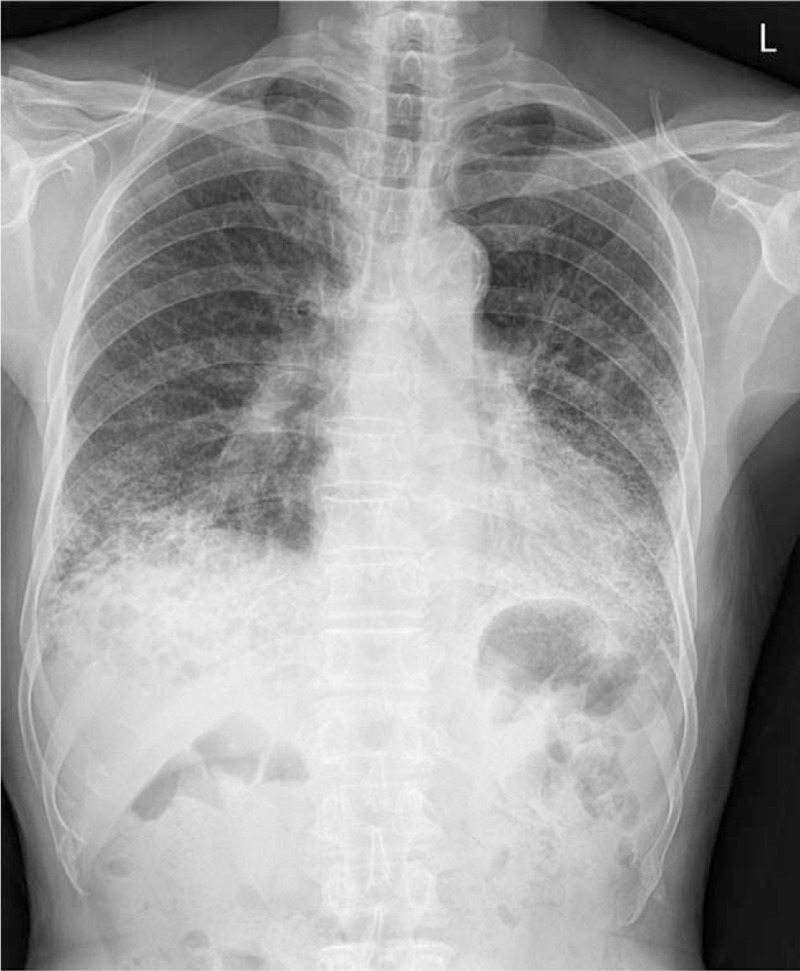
Chest X-ray showed reticulonodular infiltrations at both lung fields.
